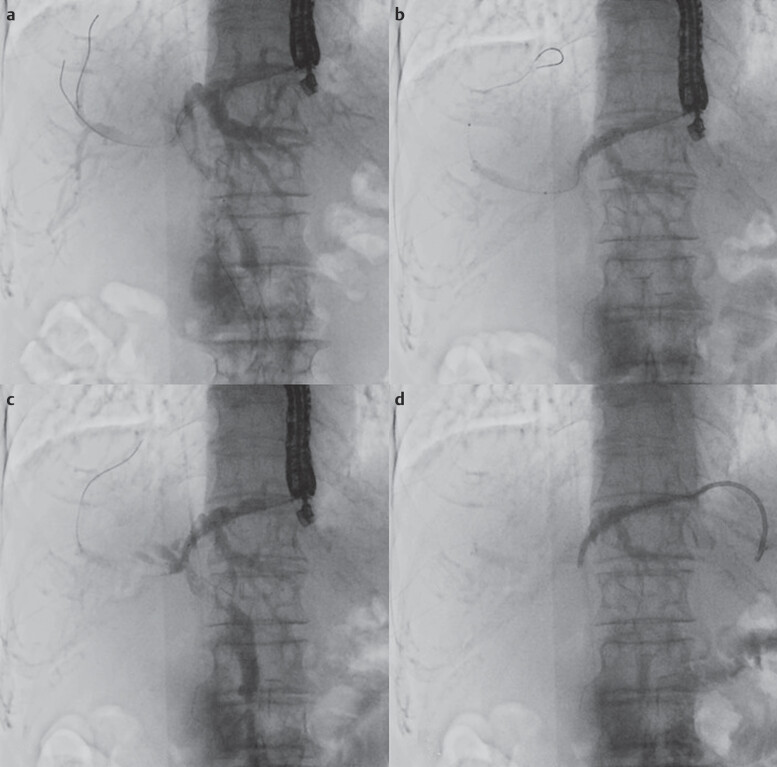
Fluoroscopic images of endoscopic ultrasound-guided
